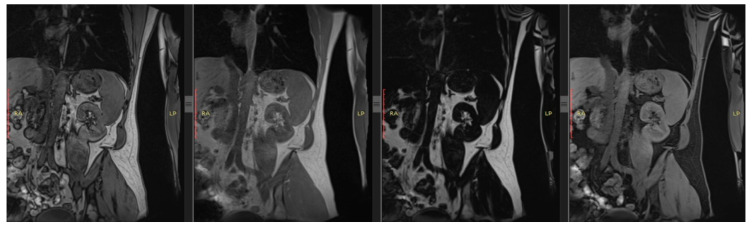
Example of kidney in T1-weighted DIXON image (from the left: in-phase image, opposed-phase image, fat only image, water only image).
